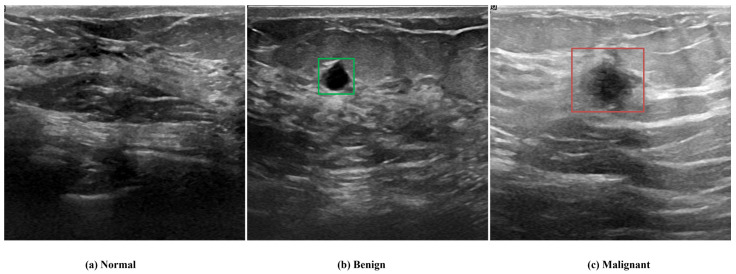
Representative ultrasound images from the BUSI dataset: (a) normal breast tissue, (b) benign lesion (outlined in green), and (c) malignant lesion (outlined in red).
